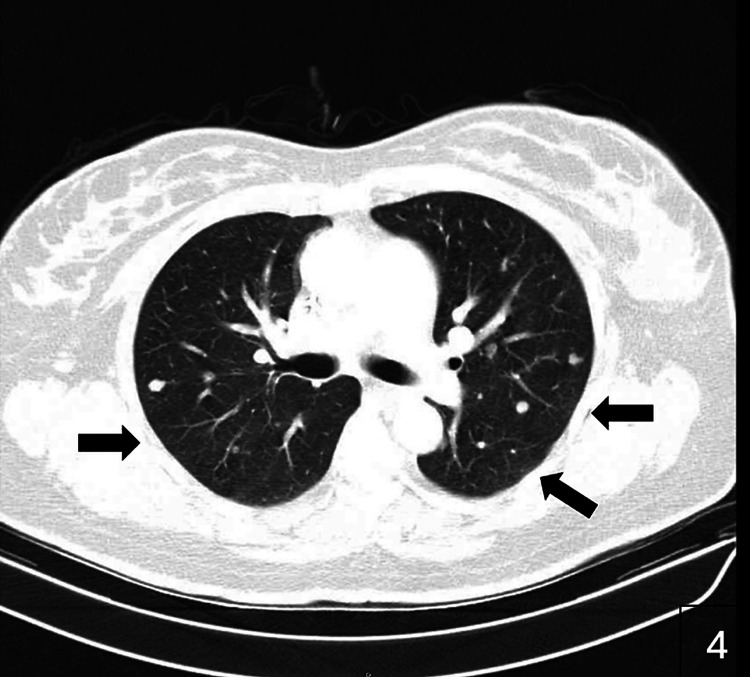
Axial CT scan showing multiple round nodules of varying sizes in both lungs, consistent with pulmonary metastases (arrows).
